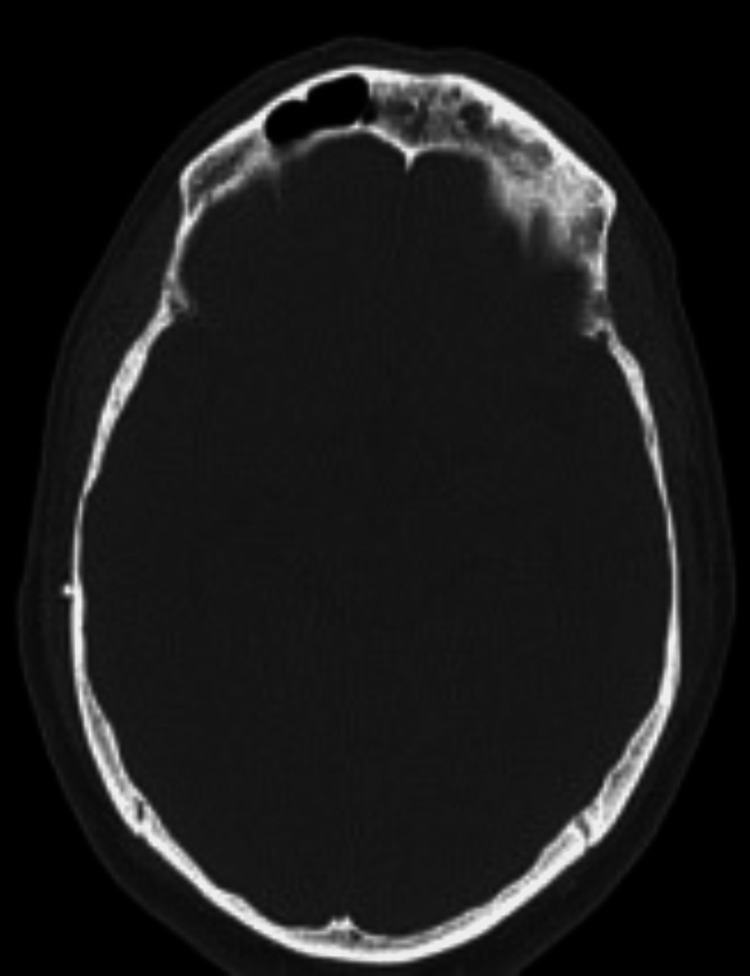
A 16-month postsurgical axial CT demonstrating progressive ossification of the left frontal sinus.
